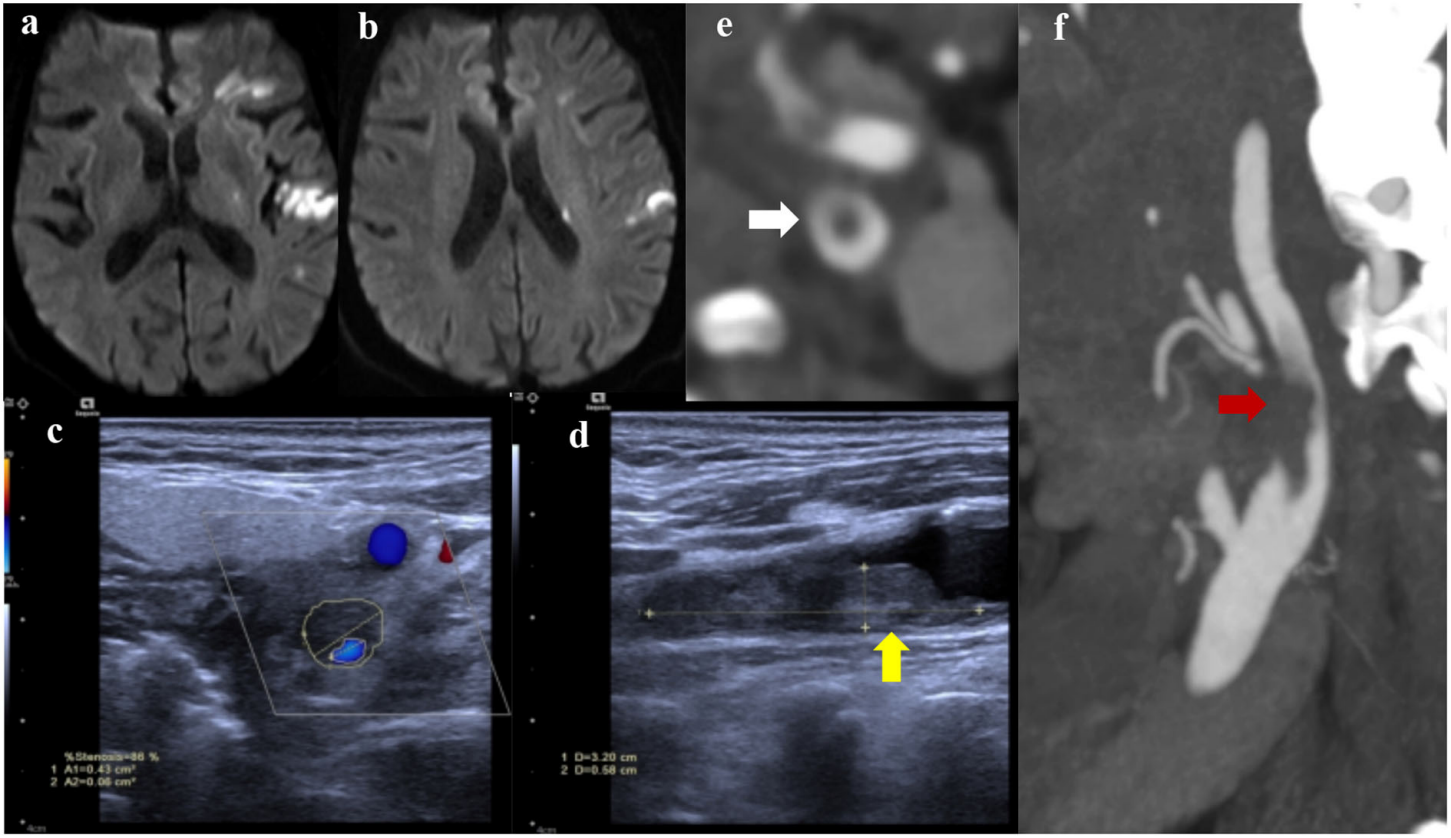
Diffusion-weighted imaging (DWI) showed multiple infarctions in the left hemisphere (a,b); Carotid ultrasound (c,d) indicated a culprit plaque with local floating thrombus (yellow arrow), resulting in obvious stenosis of the left internal carotid artery; carotid CTA (e,f) presented the floating thrombus (red arrow) with a typical cross-sectional “donut sign” (white arrow).
